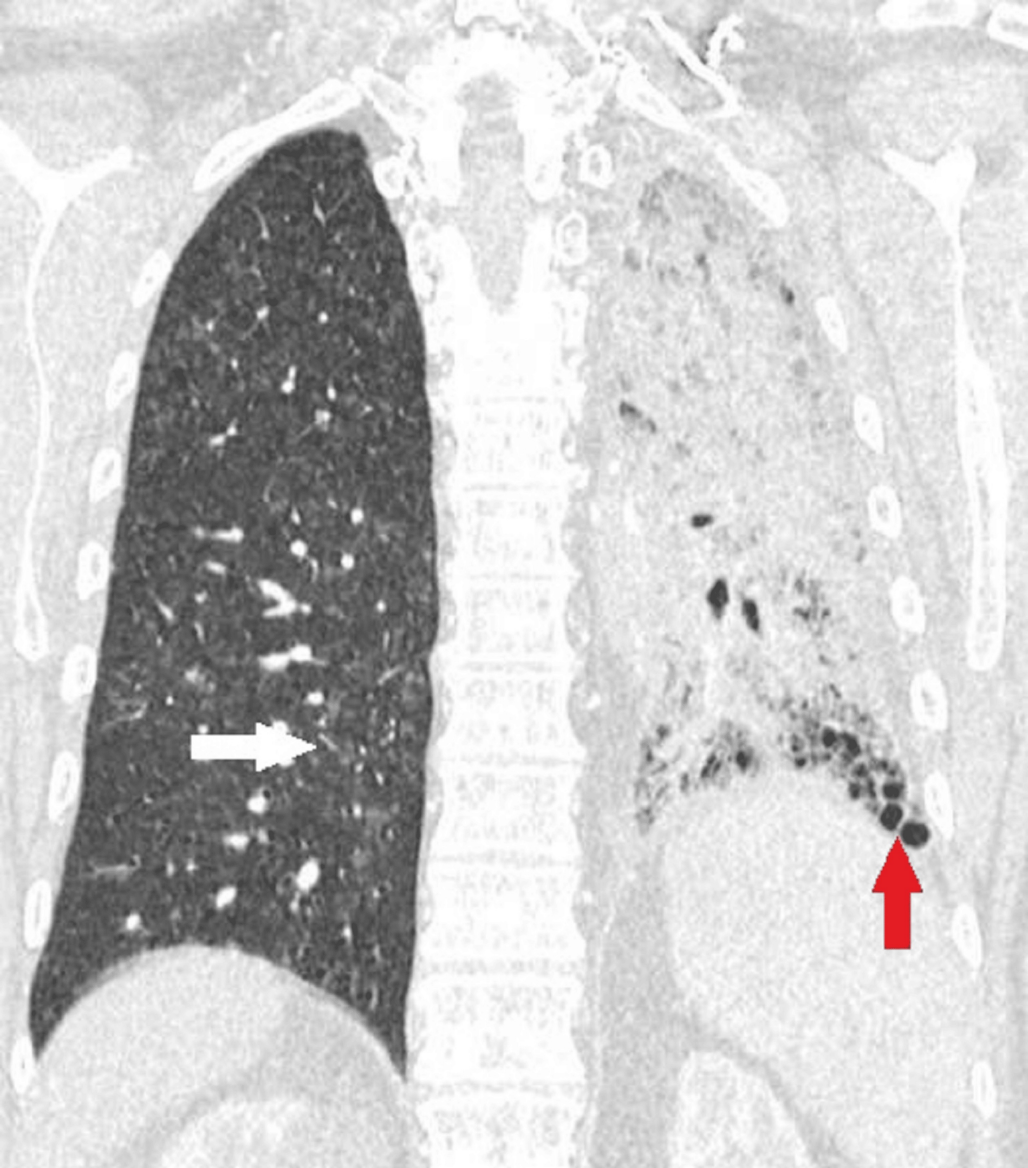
Lung window, coronal view. The CT image demonstrates decreased left lung volume relative to the right with mediastinal shift to the left. There is extensive left lung airspace consolidation with honeycombing in the left lower lobe (red arrow), as well as bronchiectasis, consistent with asymmetric pulmonary fibrosis of the left lung. On the contralateral lung (right), there are ground-glass nodules in the right middle and right lower lobes (white arrow) with small volume consolidation in the dependent right lower lobe.
